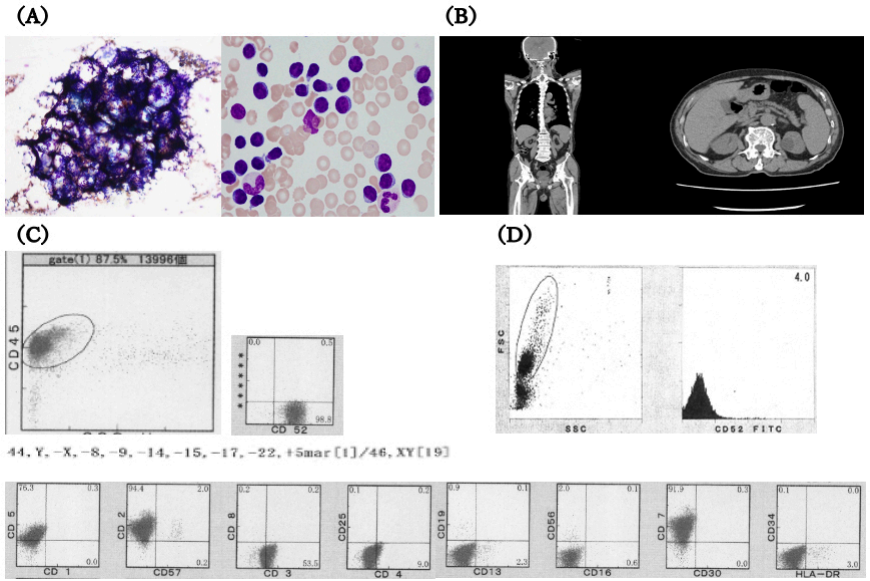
Results of laboratory testing at the first visit were as follows: (A) bone marrow smear showing cellular proliferation; (B) coronal and sagittal CT revealing splenomegaly, but no lymphadenopathy is observed; (C) flow cytometry showing tumor cells positive for CD2, CD3, CD5, CD7, and CD52 but negative for CD1, CD4, CD8, CD13, CD16, CD19, CD25, CD30, CD34, CD56, CD57, and HLA-DR. G-banding revealed complex chromosomal rearrangements. (D) CD52 in lymphocytes decreased to an extremely low level (4.0%) during relapse.
